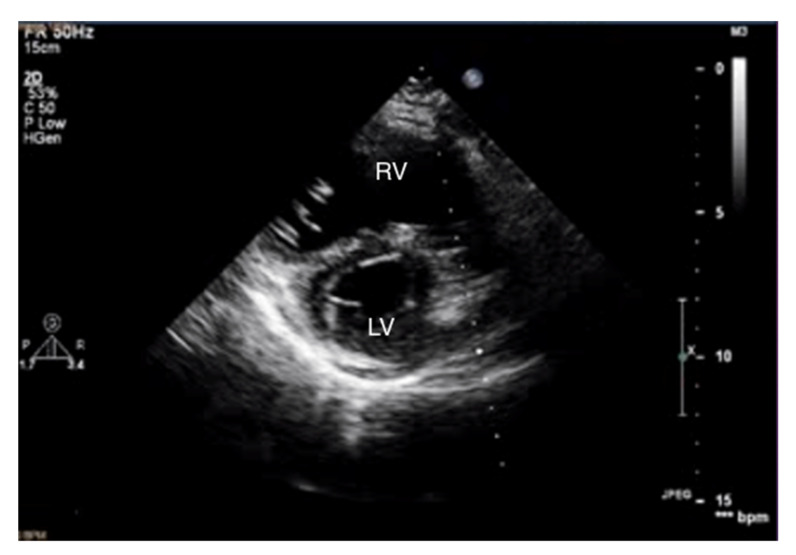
Enlarged right ventricle. 2D-TTE, parasternal short-axis view (PSAX). RV: right ventricle, LV: left ventricle.
